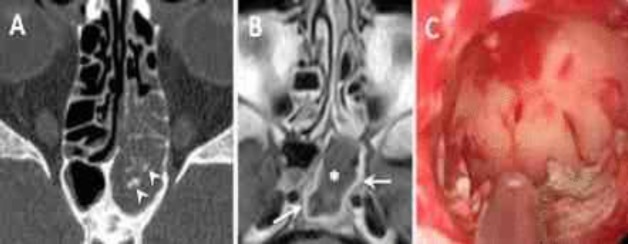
Axial (A) computed tomography scans showing left sphenoethmoidal opacification with associated multiple foci of microcalcifications (white arrowhead). There is also sclerosis of the sphenoidal sinus walls by reactive osteitis. Axial contrast-enhanced T1-weighted magnetic resonance imaging (B) showing thickened, inflamed mucosa at the periphery of mycetoma (white arrows) and intermediate signal of the fungal mass (small star). Intraoperative view of the fungal concretion in the sphenoid sinus floor (C).
